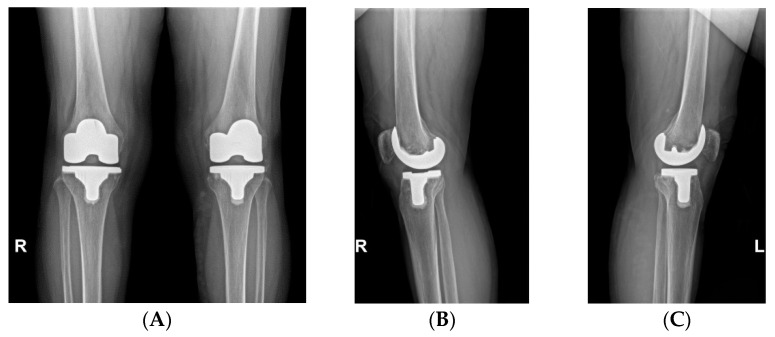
Postoperative radiographs. (A) Anteroposterior radiograph of a patient who underwent a right total knee arthroplasty (TKA) providing 1 mm increment of PE insert thickness (Exult) and a left TKA providing 2 mm increment (LOSPA). (B) Lateral radiograph of a right TKA providing 1 mm increment. (C) Lateral radiograph of a left TKA providing 2 mm increment.
